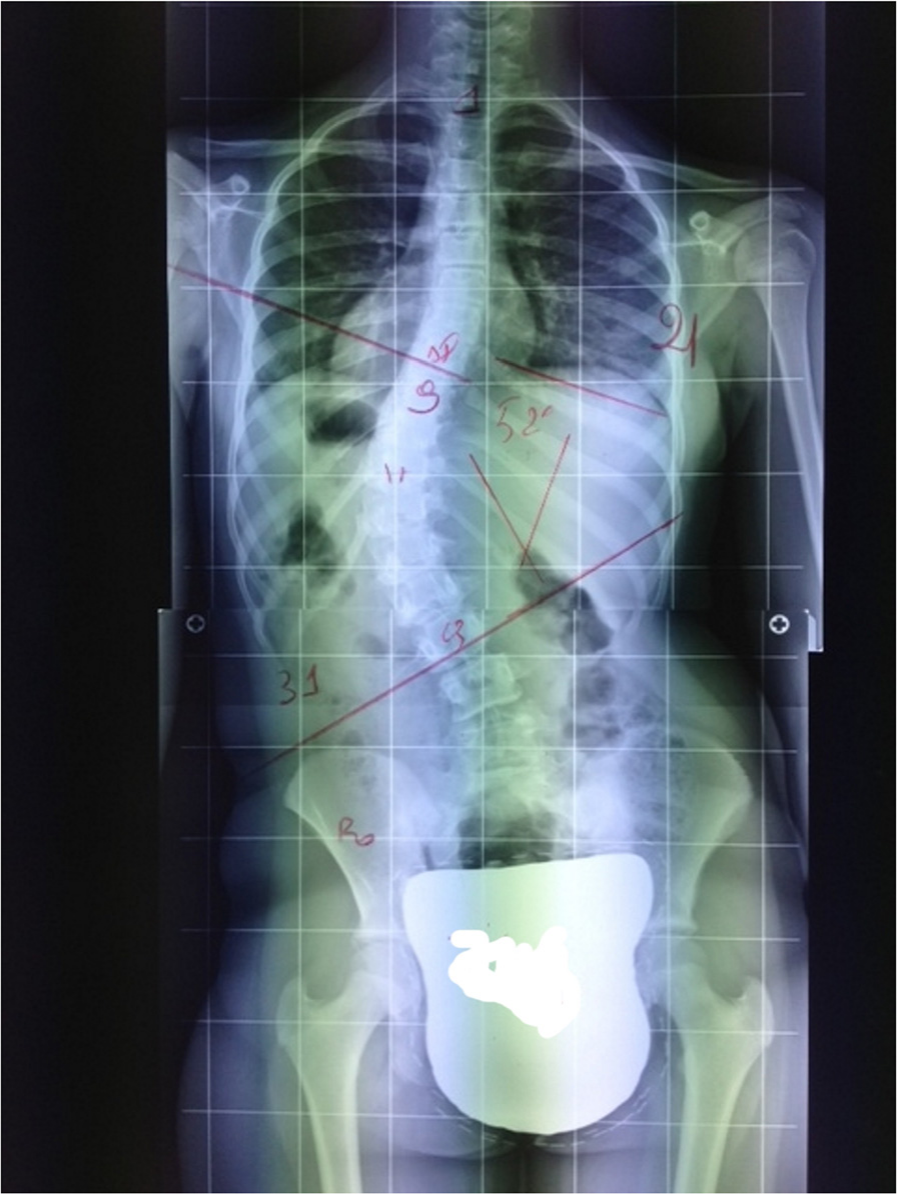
Patient 1. Postero-Anterior Spine X-ray.
